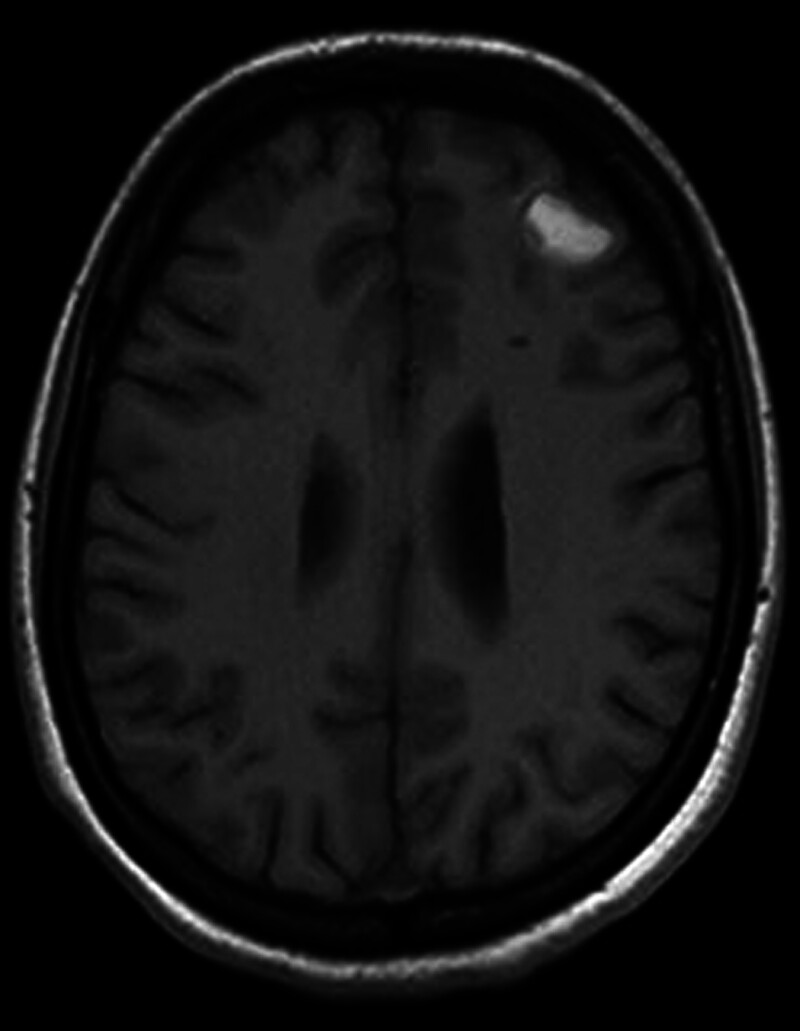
MRI indicates intracranial hemorrhage. MRI = magnetic resonance imaging.
